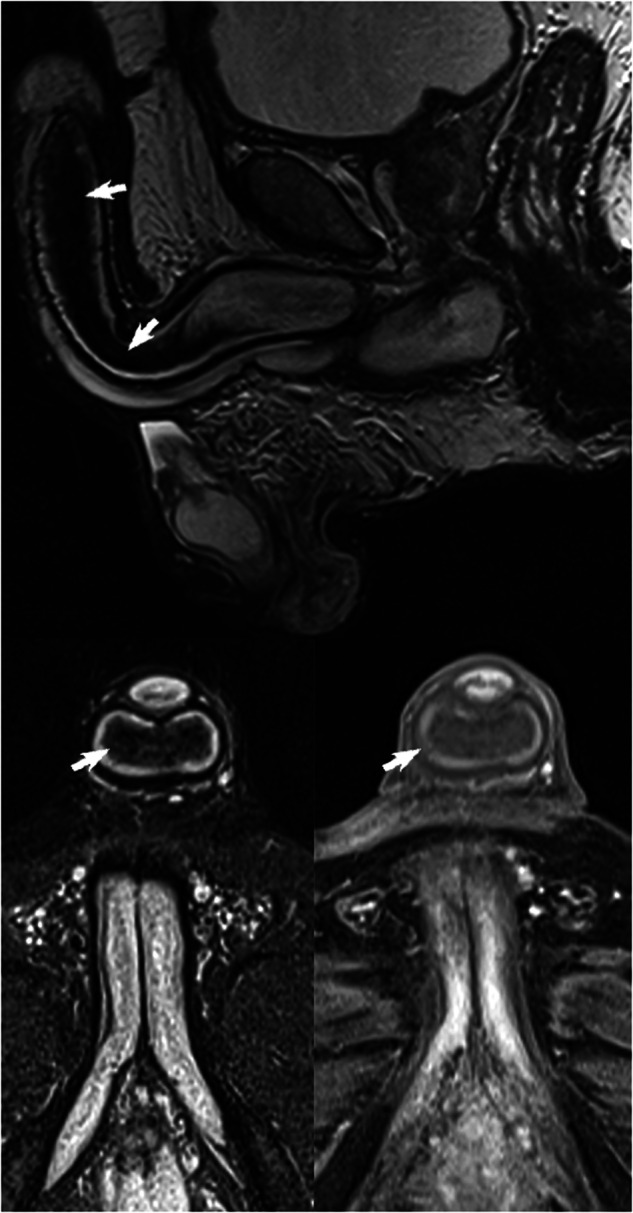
MRI in ischemic priapism. Top and bottom left image – T2 sagittal plane showing extensive low T2 signal in the central corpora cavernosa indicating necrotic/infarcted tissue. Bottom right image – T1FS post contrast at a similar level showing no central cavernosal enhancement. A thin rind of enhancement in the corpora with preserved enhancing crural tissue is seen. These appearances likely relate to dorsal penile artery branches perfusing the peripheral corporal tissue.
